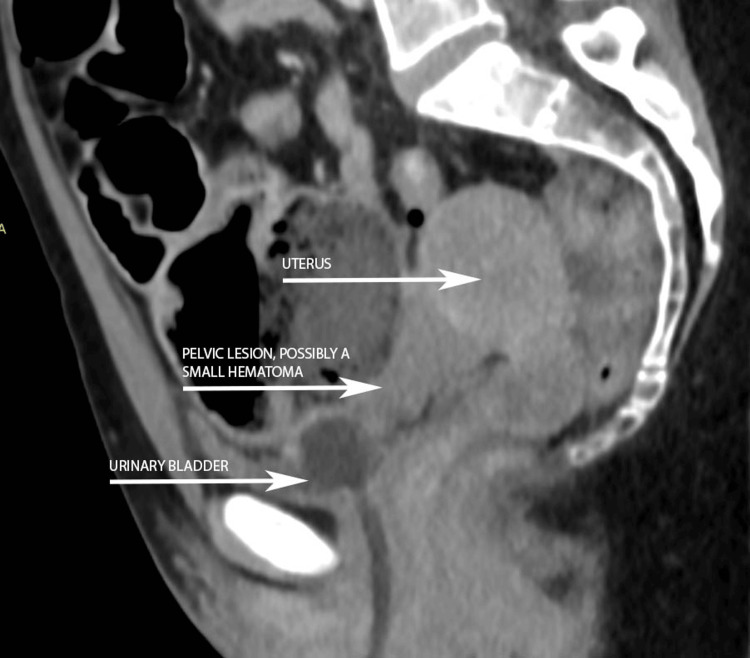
CT of the abdomen revealing a 25 × 60 mm lesion above the urinary bladder, consistent with a hematoma.
